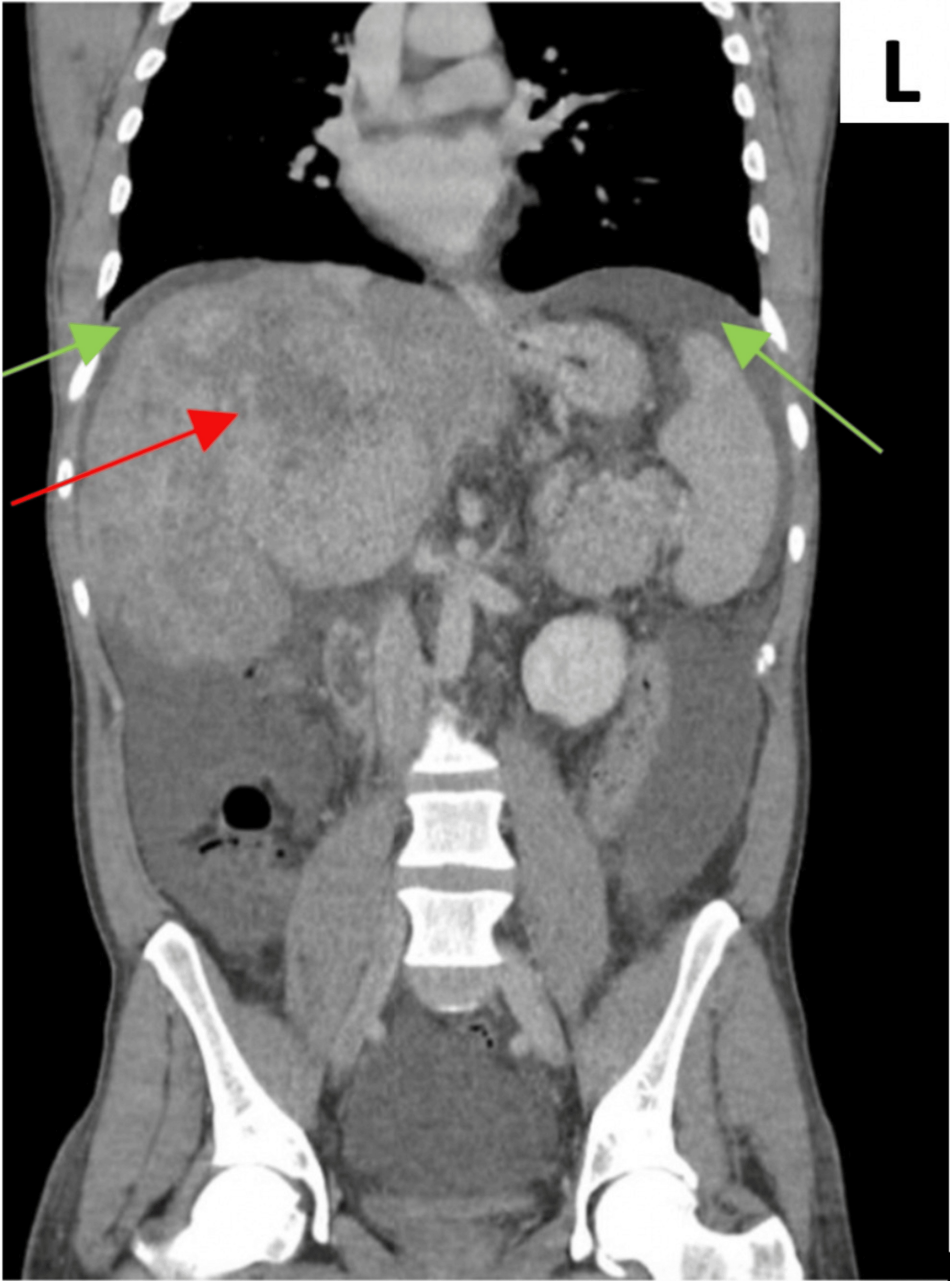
Coronal plane from a contrast-enhanced CT of the thorax, abdomen, and pelvis. The red arrow indicates a large, ill-defined mass occupying the right hepatic lobe, consistent with a possible malignant lesion. Green arrows highlight small bilateral pleural effusions. Although not shown in these images, additional findings included low-density cystic lesions within the spleen and the presence of ascites. No enlarged abdominal or pelvic lymph nodes were identified. ‘L’ indicates the left side of the body.
